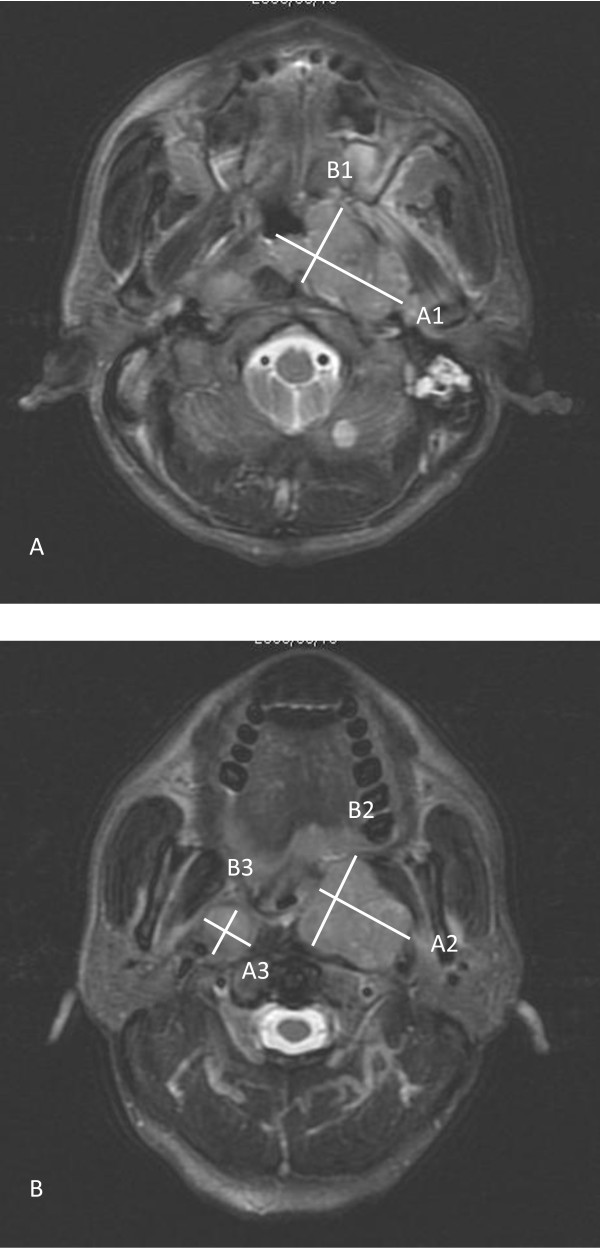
T2-weighted postcontrast MR image in the axial plane. The bidimensional measurement of primary tumor and retropharyngeal nodes (BDMprn) was obtained by summation of multiplying the maximum diameter by the greatest measurement perpendicular to it in nasopharyngeal tumor (A) and retropharyngeal nodes (B). BDMprn (cm^2^) = A1 × B1 + A2 × B2 + A3 × B3.
